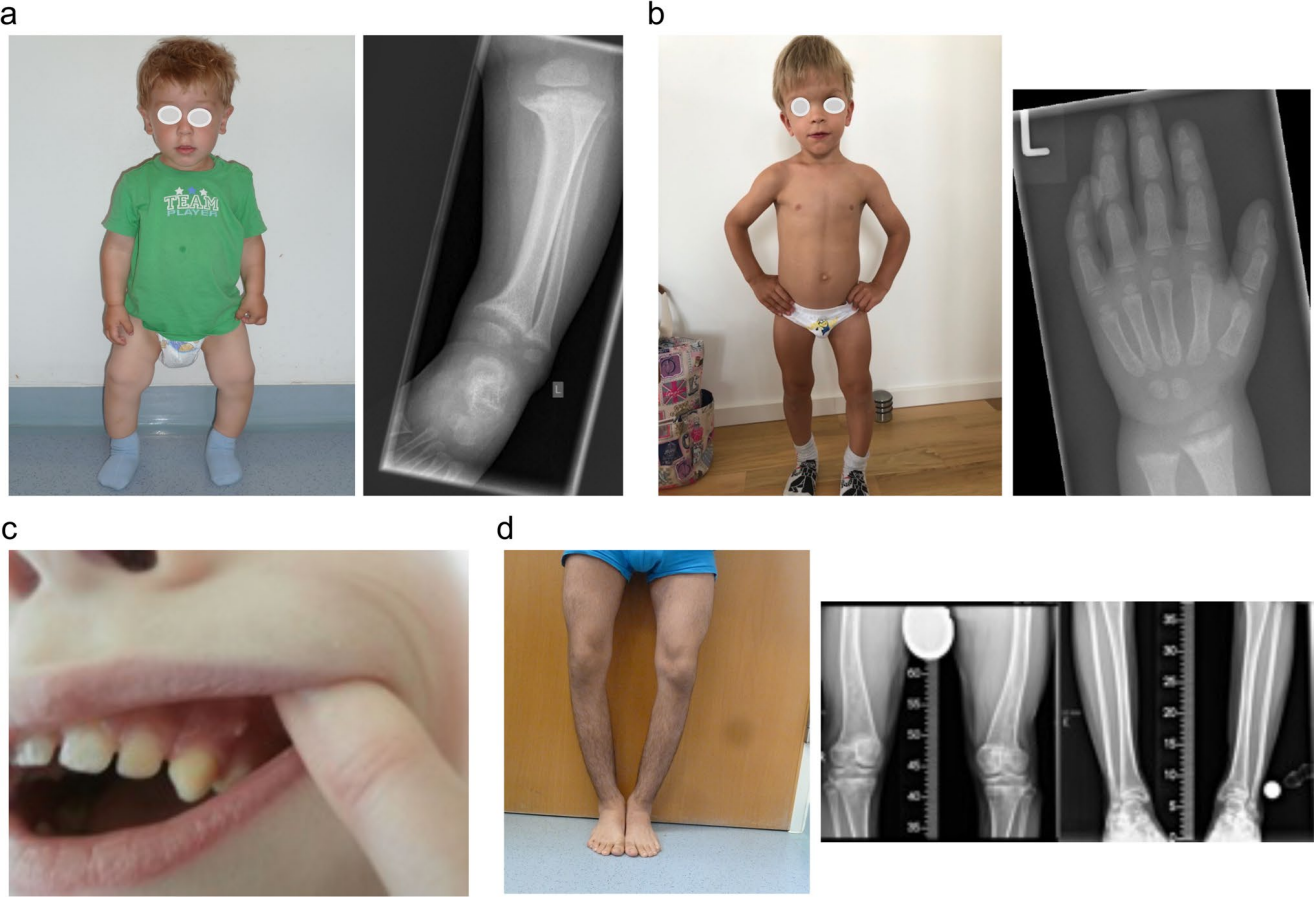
Clinical features of phosphopenic rickets. (a) 2-year-old boy diagnosed with X-linked hypophosphatemia (XLH) at the age of 2 years, presenting with disproportionate short stature (–2.3 SD score), genu vara, and widening of growth plates and metaphyseal fraying on X-rays. (b) 3-year-old patient with XLH started on treatment with active vitamin D and phosphate at the age of 2 years showing disproportionate short stature (height, –2.4 SD score), frontal bossing, dolichocephalus and mild signs of rickets on X-ray. (c) Dental abscess on an apparently healthy tooth in a child with XLH. (d) 16-year-old boy with autosomal-recessive hypophosphatemic rickets type 2 (ARHR2) showing genu vara and mild ricketic signs on X-ray. Figure 3c reproduced with permission from Haffner and Linglart [110]
